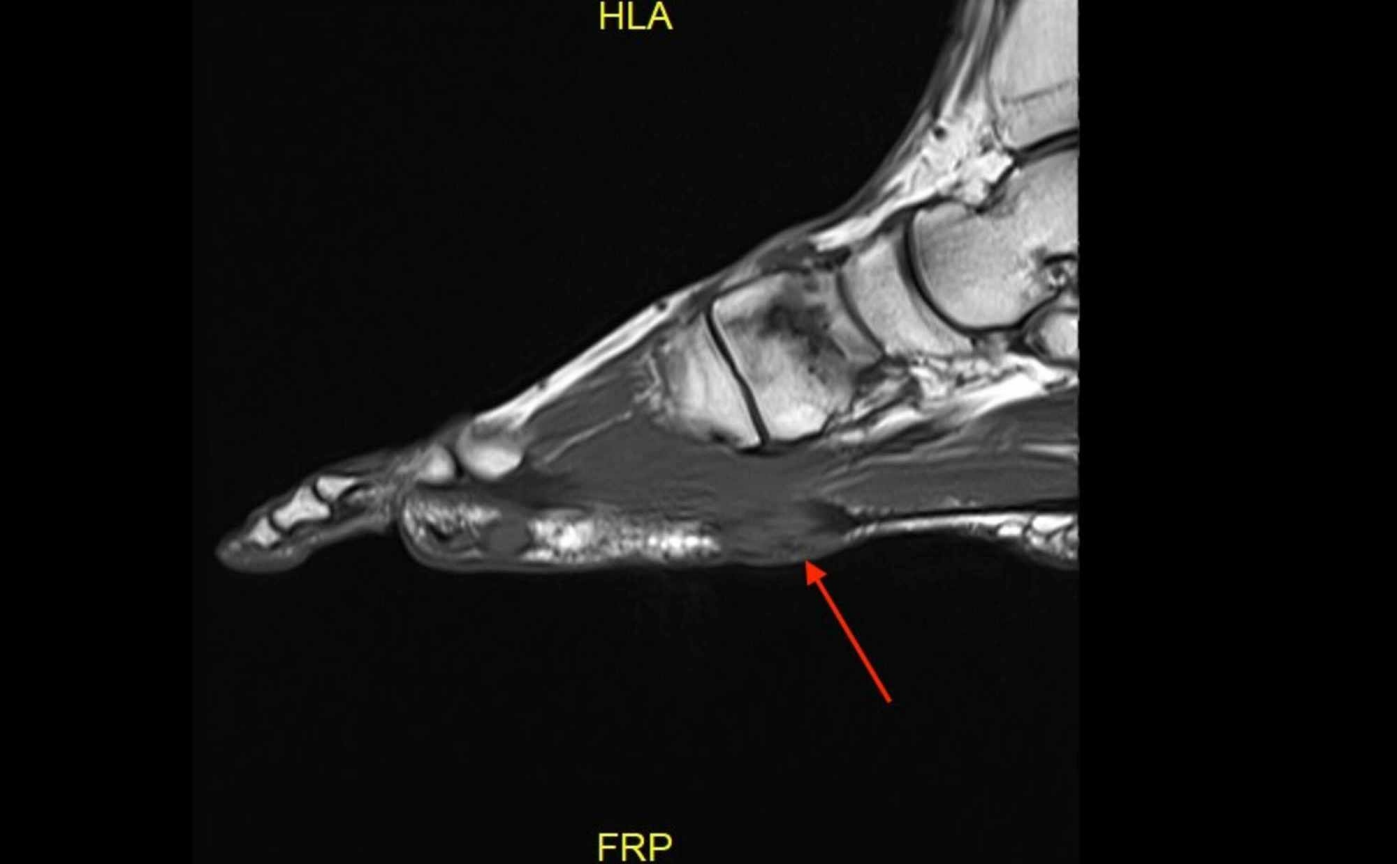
Case 2 MRI showing the sagittal T1 weighted image demonstrating a plantar fibroma of the first tarsometatarsal joint.
